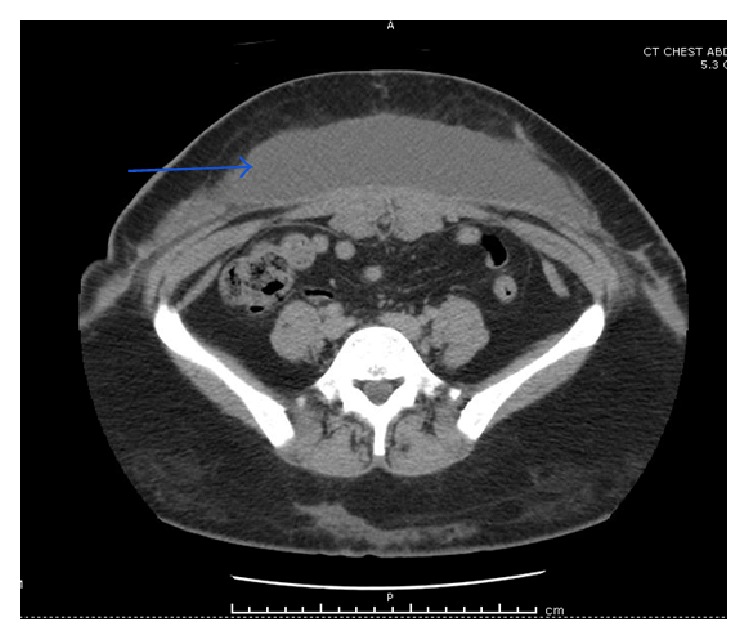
CT abdomen and pelvis with intravenous contrast showing large subcutaneous collection in the anterior abdominal wall (blue arrow) suggestive of abscess.
